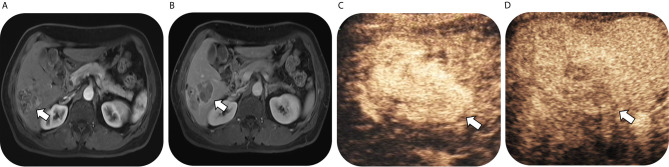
Images in a 54-year-old man with chronic hepatitis B virus infection and pathological confirmed combined hepatocellular cholangiocarcinoma, which was correctly classified as LR-M on CEUS but mistaken as LR-5 on gadopentetate-enhanced MRI. T1-weighted image shows a 53-mm nodule in hepatic segment VI with arterial phase hyperenhancementand (arrow) in (A) arterial phase followed by enhancing capsule (arrow) and non-rim washout in (B) delayed phase. Contrast-enhanced US image shows a 64-mm nodule with heterogeneous hyperenhancement (not rim or peripheral discontinued globular enhancement) (arrow) in (C) arterial phase (timer, 00:19) followed by early washout (arrow) in (D) portal phase (timer, 00:51) and mild washout in delayed phase.
